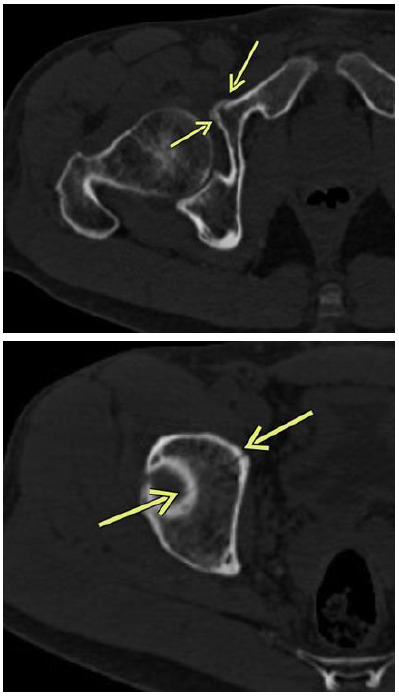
Case 1: Computed tomography scan of the right hip 2 days post injury demonstrating a fracture of the anterior column and extension into the roof of the acetabulum
